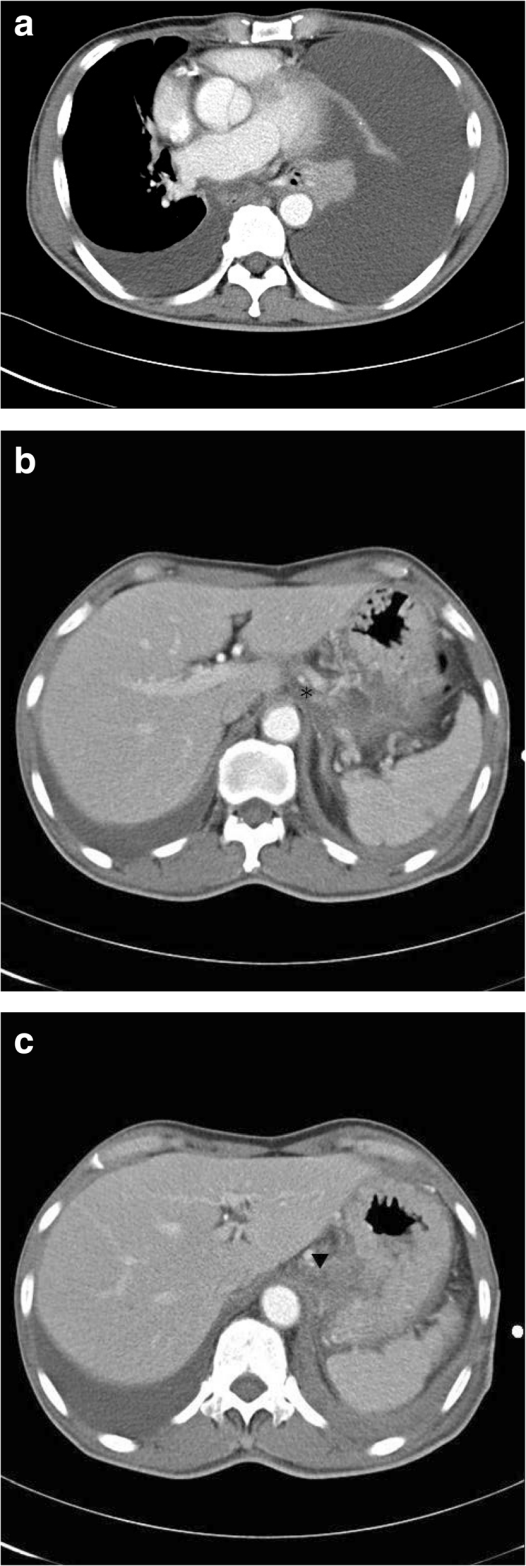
Computed tomography scans of the chest and abdomen. a The computed tomography scan of the chest reveals left massive hydropneumothorax with collapse of most of the left lung and shifting of the mediastinum to the right side. b The *asterisk* shows the pseudocyst in the pancreatic tail. c The *inverted triangle* shows the pseudocyst in the pancreatic tail
